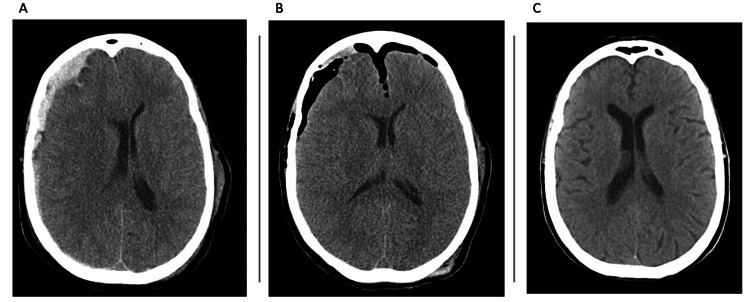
Preoperative and postoperative computed tomography scans of the same patient with subdural hematoma (A) Axial view of a computed tomography (CT) scan depicting an acute subdural hematoma of a patient from our series. (B) Axial view of a CT scan of the same patient in the immediate postoperative phase. (C) Axial view of a CT scan of the same patient during a follow-up visit four weeks after surgery.
